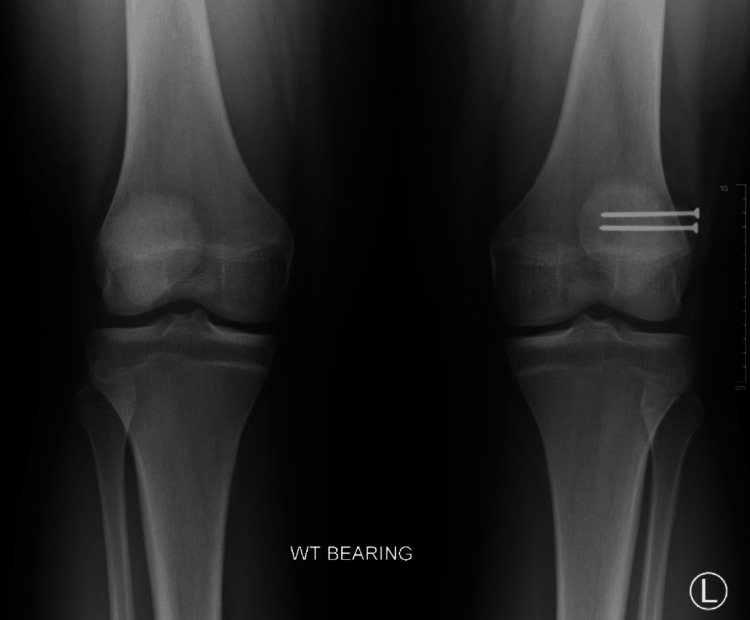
Six-month postoperative X-ray bilateral knee AP weight-bearing view AP: Anteroposterior.
